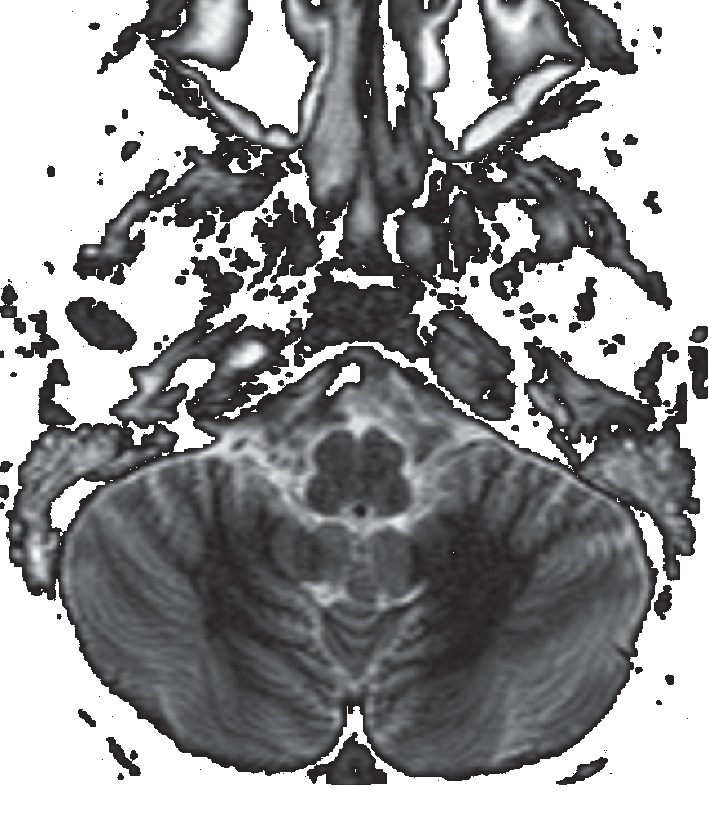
Axial STIR sequence magnetic resonance imaging (MRI) shows a diffuse bilateral inflammatory involvement of middle ear-mastoid with extension of the inflammatory process to petrous apex on the left side.
